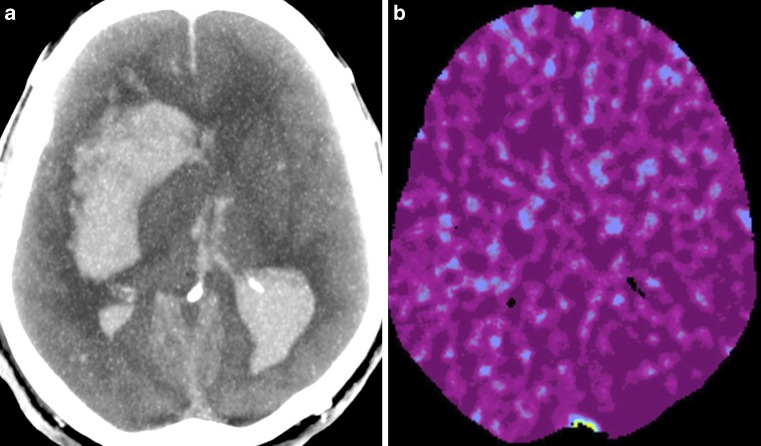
Results of CTA (a) and CTP (b) in the patient diagnosed with brain death. The CTA shows absence of filling of intracranial arteries and veins and was classified as positive, i. e. consistent with the diagnosis of BD. CTP reveals perfusion values below the thresholds for non-viable tissue and, like CTA, was interpreted as positive, i. e. confirming the diagnosis of BD
